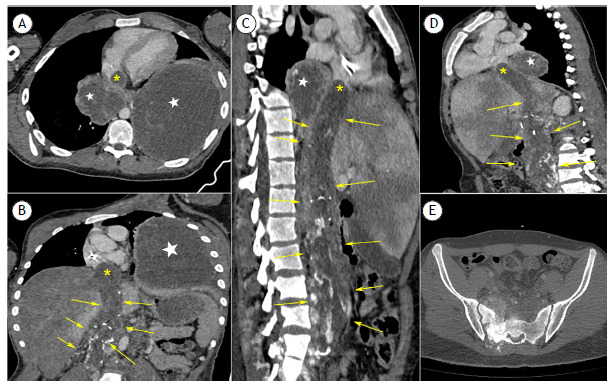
Intravenous contrast-enhanced CT scans of the chest, abdomen, and pelvis, with axial (in A), coronal (in B), and sagittal (in C and D) reconstructions of the thoracoabdominal region, as well as axial CT scan of the pelvis (in E), showing a heterogeneous mass with coarse foci of calcification originating in the pelvic region and extending superiorly through the inferior vena cava (yellow arrows), reaching the right atrium (yellow asterisks). Heterogeneous masses are also present in the right posteroinferior mediastinal region and left lower lobe (in A; white stars), in addition to a mass projecting from the right atrium (yellow asterisks). In E, a mixed lytic and sclerotic lesion is seen on the right side of the sacrum.
